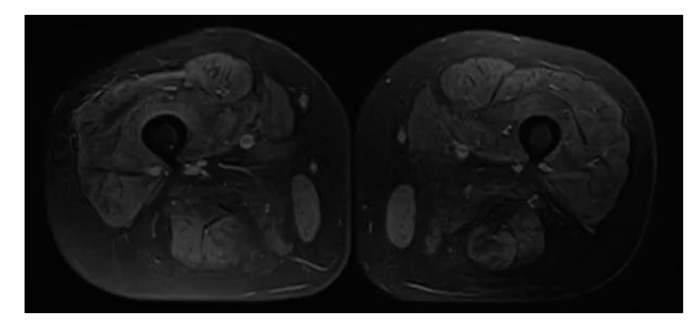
Axial fat-saturated proton density-weighted MRI scan with diffuse decrease of signal intensity from all muscles of the both shins.
